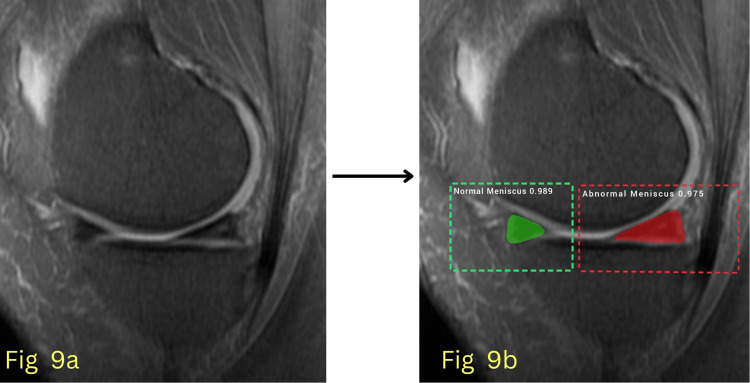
(a) Input MRI image; (b) Segmented image showing a normal anterior horn and an abnormal posterior horn of the meniscus.
